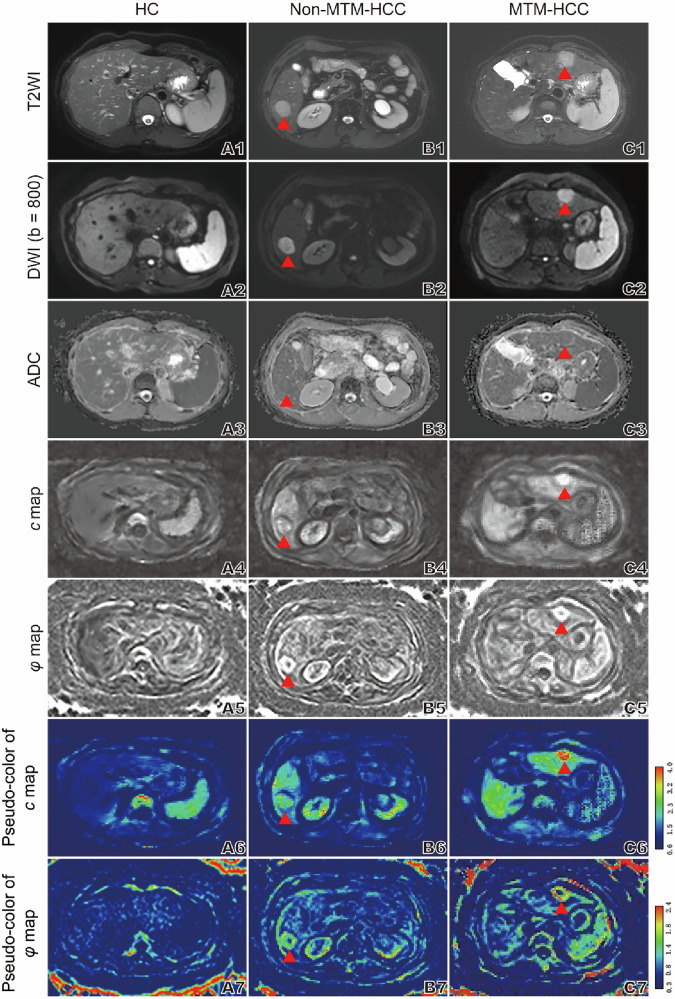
Representative imaging: Row 1: axial T2-weighted images; Row 2: axial diffusion-weighted images (DWI) at b value of 800 s/mm^2^; Row 3: corresponding apparent diffusion coefficient (ADC) maps; Row 4: axial MRE; Rows 5–7: *c* (stiffness) (Row 5) and *φ* (viscosity) (Row 6) maps and corresponding pseudo-color maps (Row 7) for three participants. A1–A7 Images in a healthy control subject (HC). B1–B7 Images in a participant with non-MTM-HCC in segment VI of liver (*c* = 1.918 m/s; *φ* = 1.345 radian). C1–C7 Images in a participant with MTM-HCC in segment II of liver (*c* = 3.585 m/s; *φ* = 1.608 radian). The red triangles mark the HCC tumor
